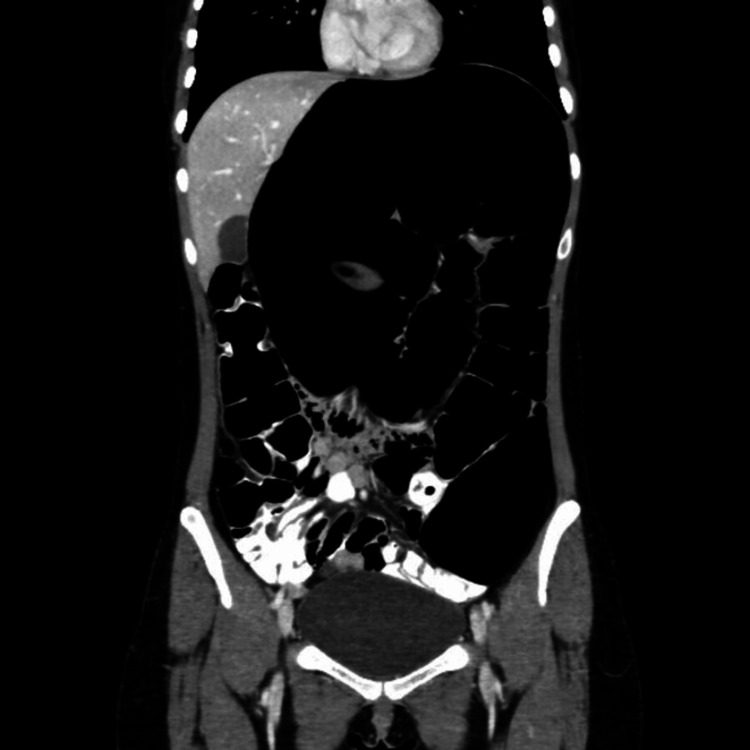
CT abdomen with IV and rectal contrast showing omega sign, suggesting sigmoid volvulus
